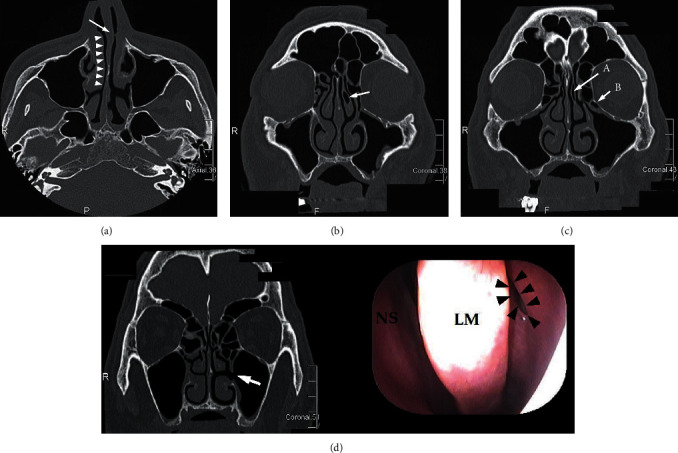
Preoperative CT: (a) left nasal septal deviation (arrow) and swelling of right inferior turbinate (arrowheads); (b) left concha bullosa of middle turbinate (arrow); (c) the narrow of left maxillary sinus ostium owing to the left concha bullosa of middle turbinate (arrow A) and left Haller cell (arrow B). (d) Left accessory ostium of maxillary sinus (arrow in CT (left) and arrowheads in nasal endoscopic examination (right). NS, nasal septum; LM, left middle turbinate.
